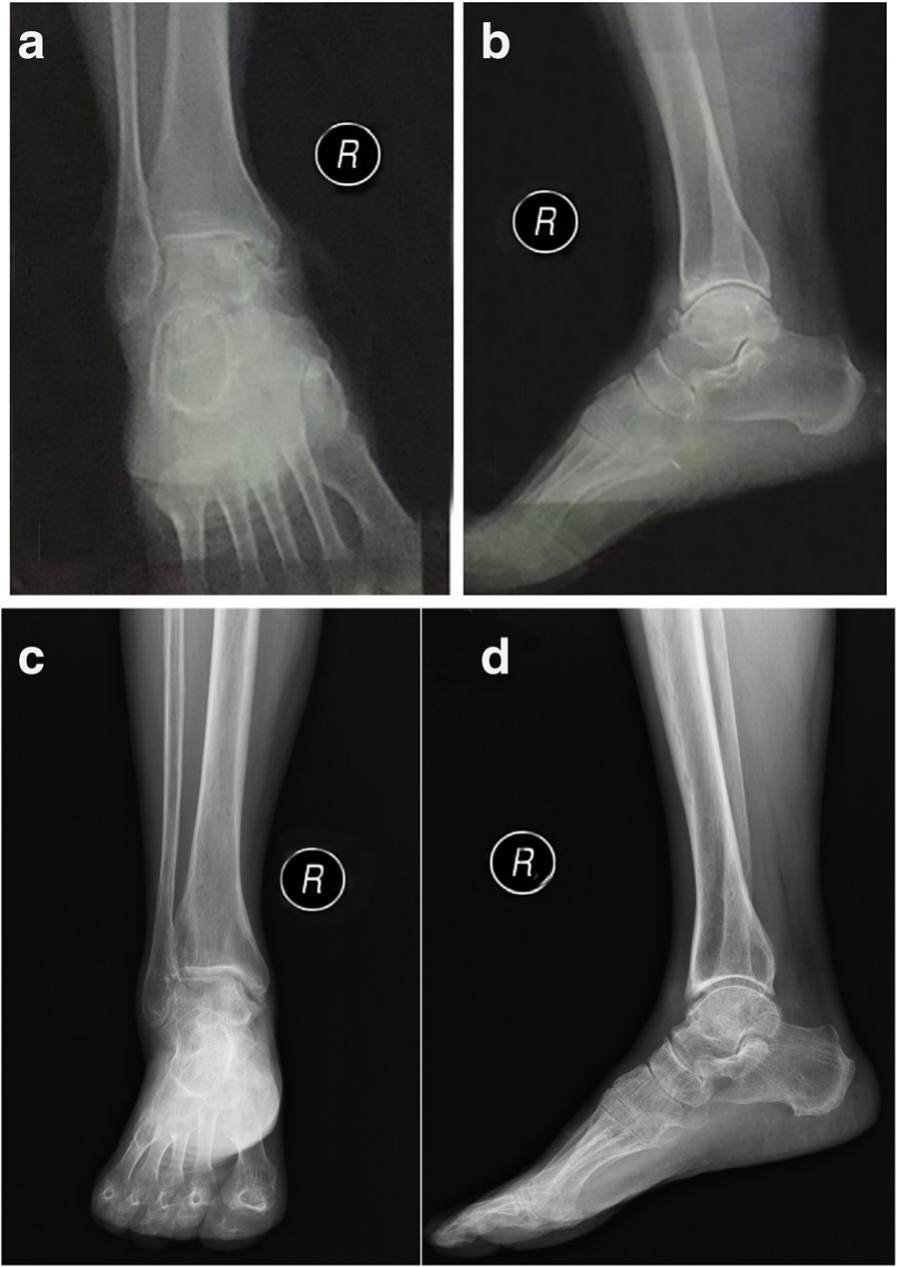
The typical radiographic views of the ankle before and after distraction arthroplasty combined with arthroscopic microfracture. a, b Preoperative anteroposterior and lateral radiographic views of the ankle suggested post-traumatic ankle arthritis characterized by osteophyte formation and obliteration of the joint space. c, d At the final follow-up of 28 months postoperatively, anteroposterior and lateral radiographic views of the ankle demonstrated joint-space enlargement and absence of osteophyte
